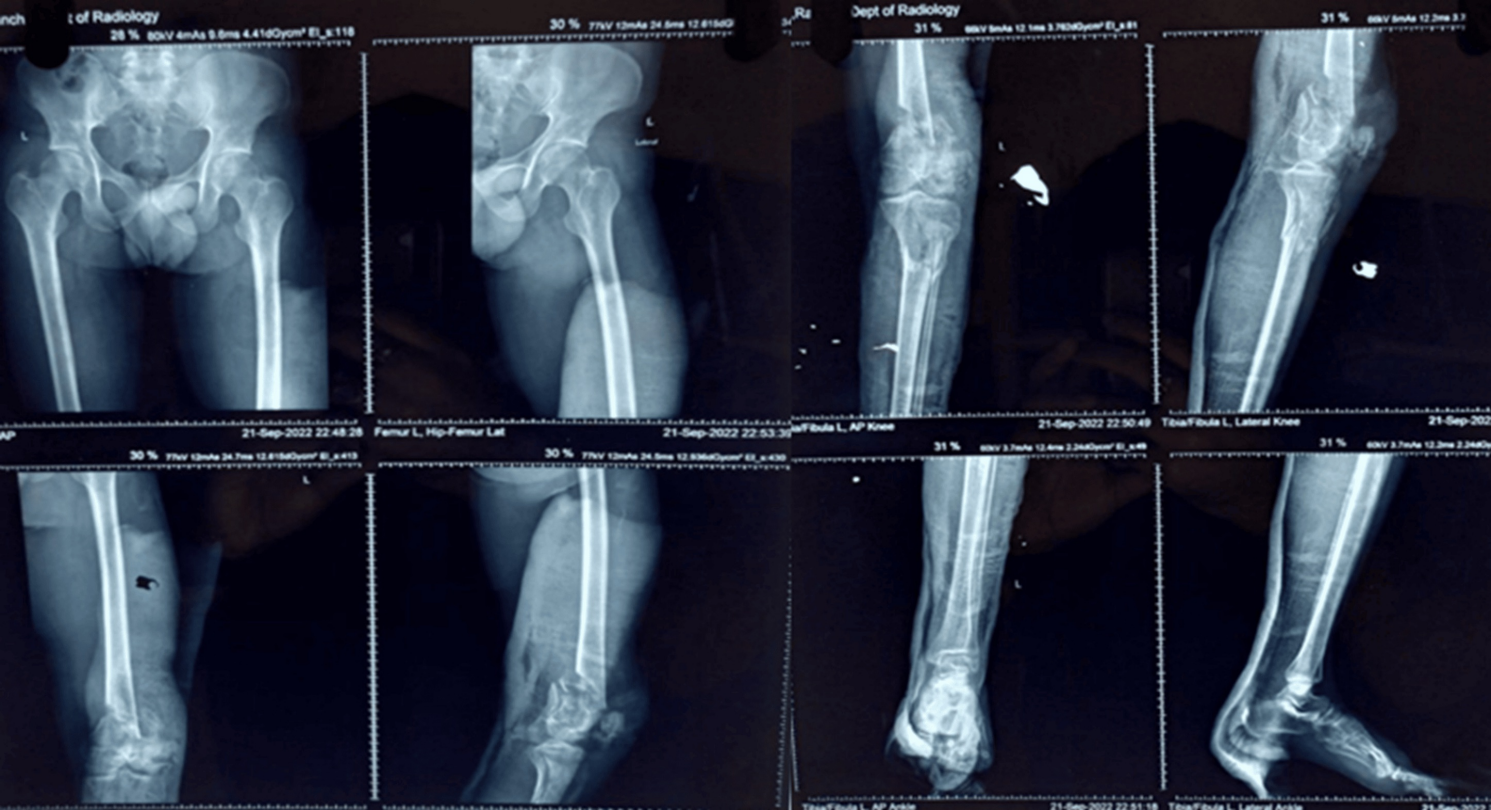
Preoperative radiograph showing a fracture of the distal end of the femur and the proximal tibia (both intra-articular) indicating a type IIc floating knee injury
